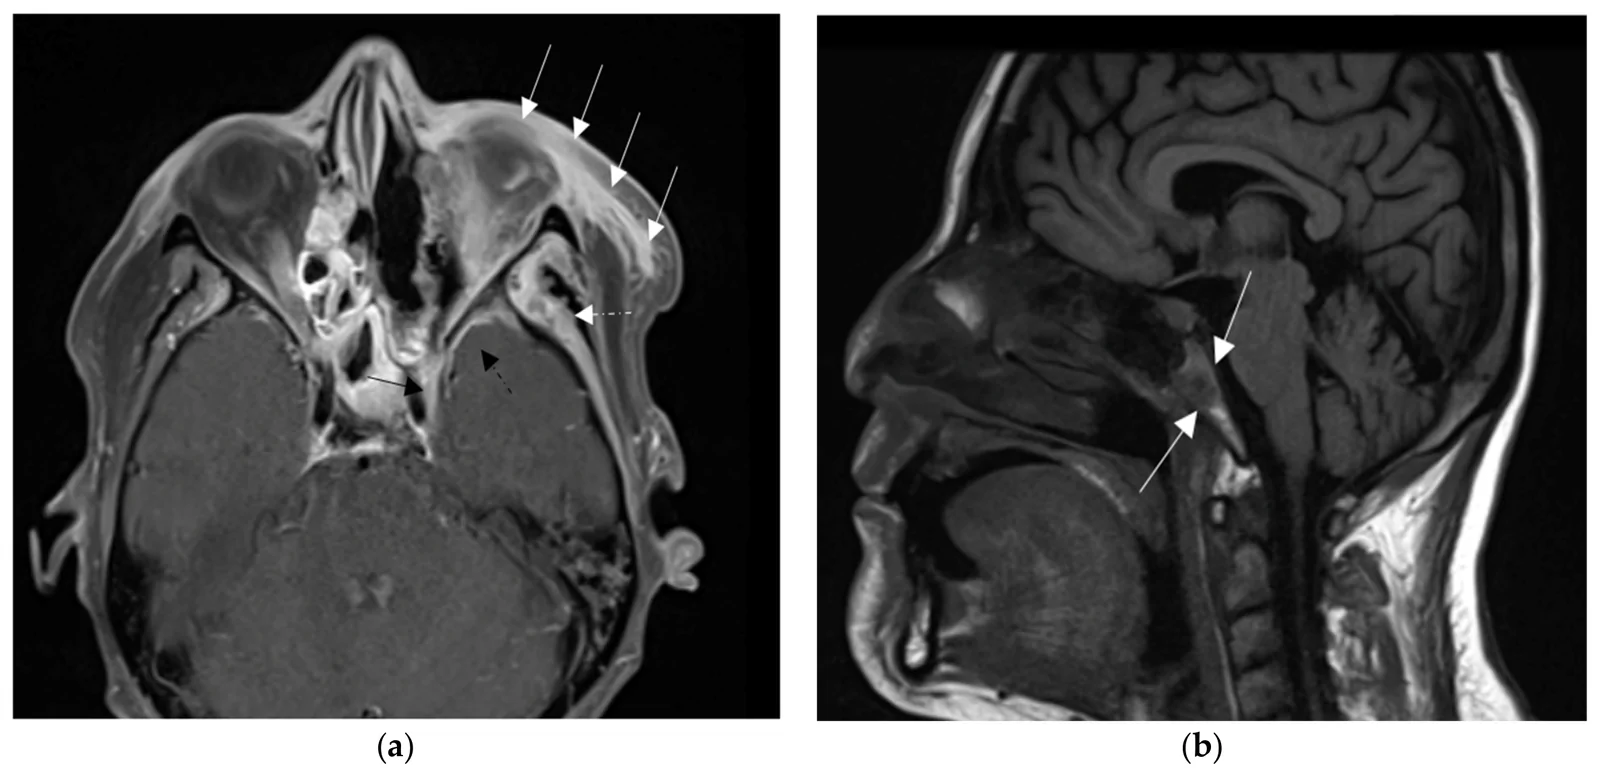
(a) Axial T1 fat-saturated post-gadolinium injection MRI sequence of the head. There is diffuse cellulitis of the left pre-septal periorbital soft tissue (white arrows) and diffuse myositis in the left upper lateral face and left suprazygomatic muscle (dashed white arrow). There is subtle increased enhancement of the left cavernous sinus (black arrow) and subtle pachymeningeal enhancement (black dashed arrow). (b) Sagittal T1 non-fat-saturated pre-gadolinium injection MRI sequence of the head and neck demonstrating low signal intensity in the clivus with thickening of the nasopharynx concerning for early acute osteomyelitis (white arrows).
